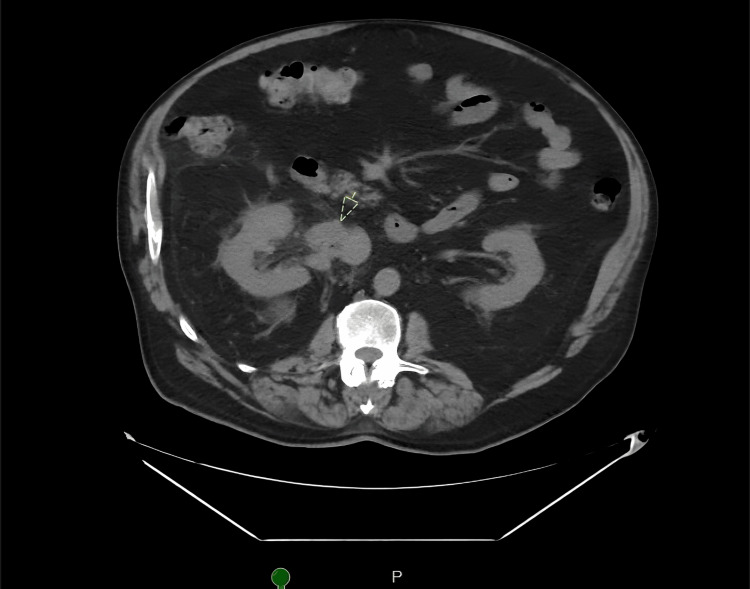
CT Abdomen/Pelvis Image Showing the Right Vein Tumor and IVC Thrombus IVC: Inferior vena cava
